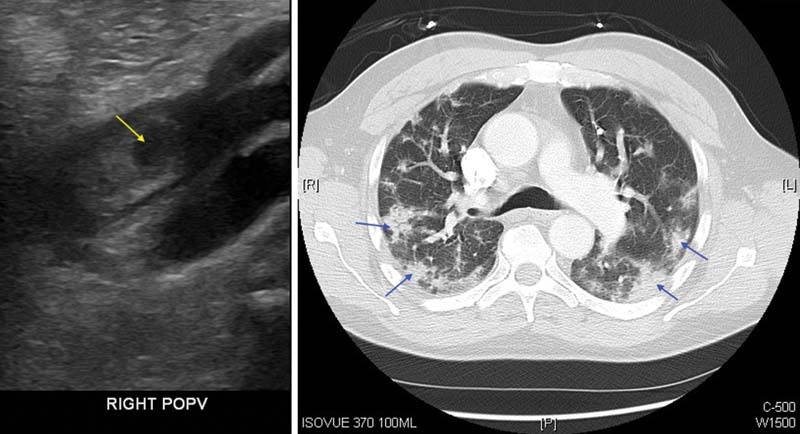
Left panel shows DVT on ultrasonography (yellow arrow); right panel shows CT with ground glass opacities (blue arrows). CT, computed tomography; DVT, deep vein thrombosis.
